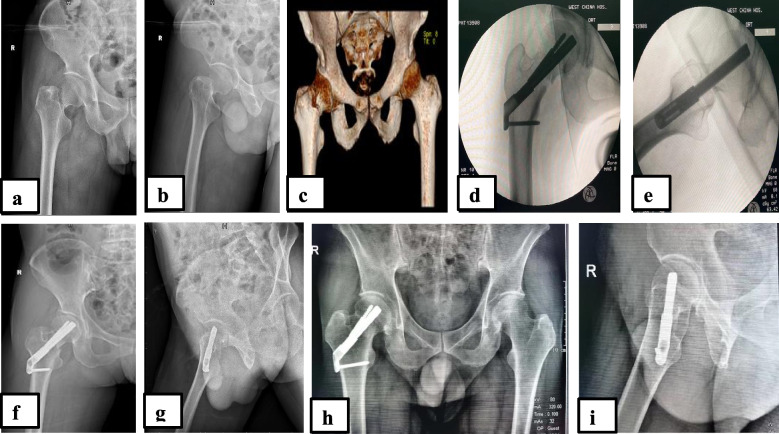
A 60 years old male patient with right femoral neck fracture (Garden type III), managed operatively with internal fixation using Femoral Neck System 24 h after injury. Preoperative radiographs anteroposterior view (a), lateral view (b) and 3D CT scan (c); intraoperative fluoroscopic anteroposterior view (d), lateral view (e); immediate postoperative radiographs anteroposterior view (f) and lateral view (g); postoperative 13 months radiographs anteroposterior view (h) and lateral view (i)
